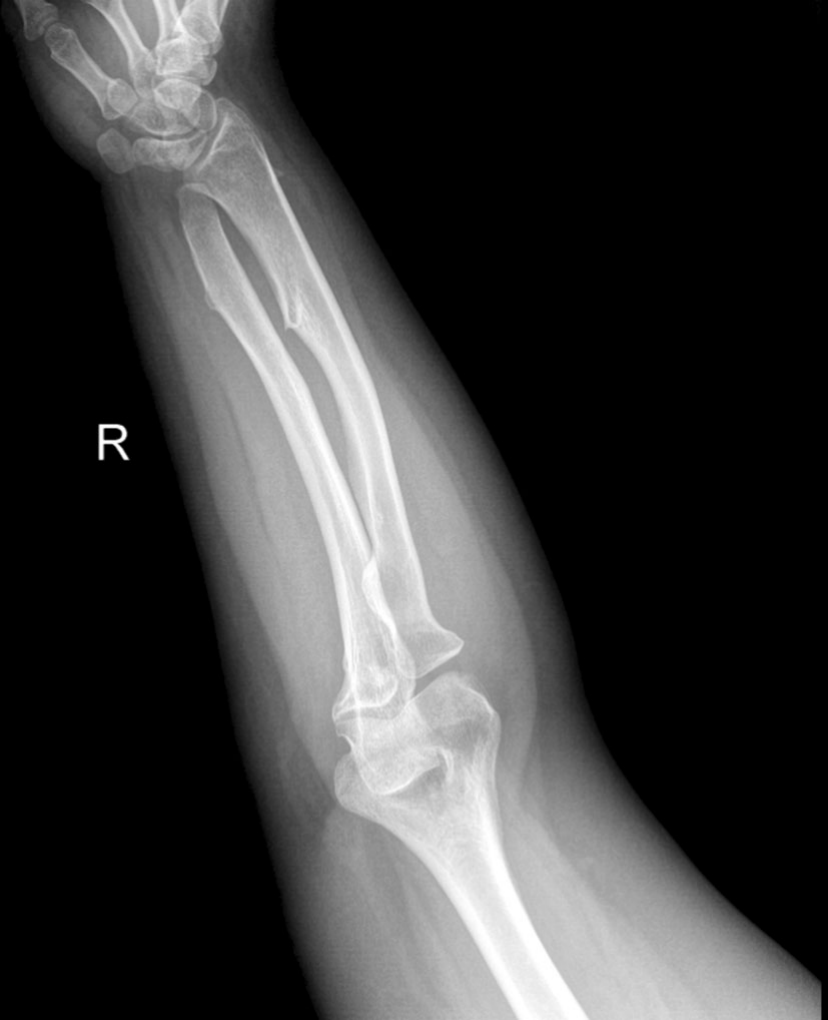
Anteroposterior radiograph of a forearm at skeletal maturity: the radial head appears in the correct position
